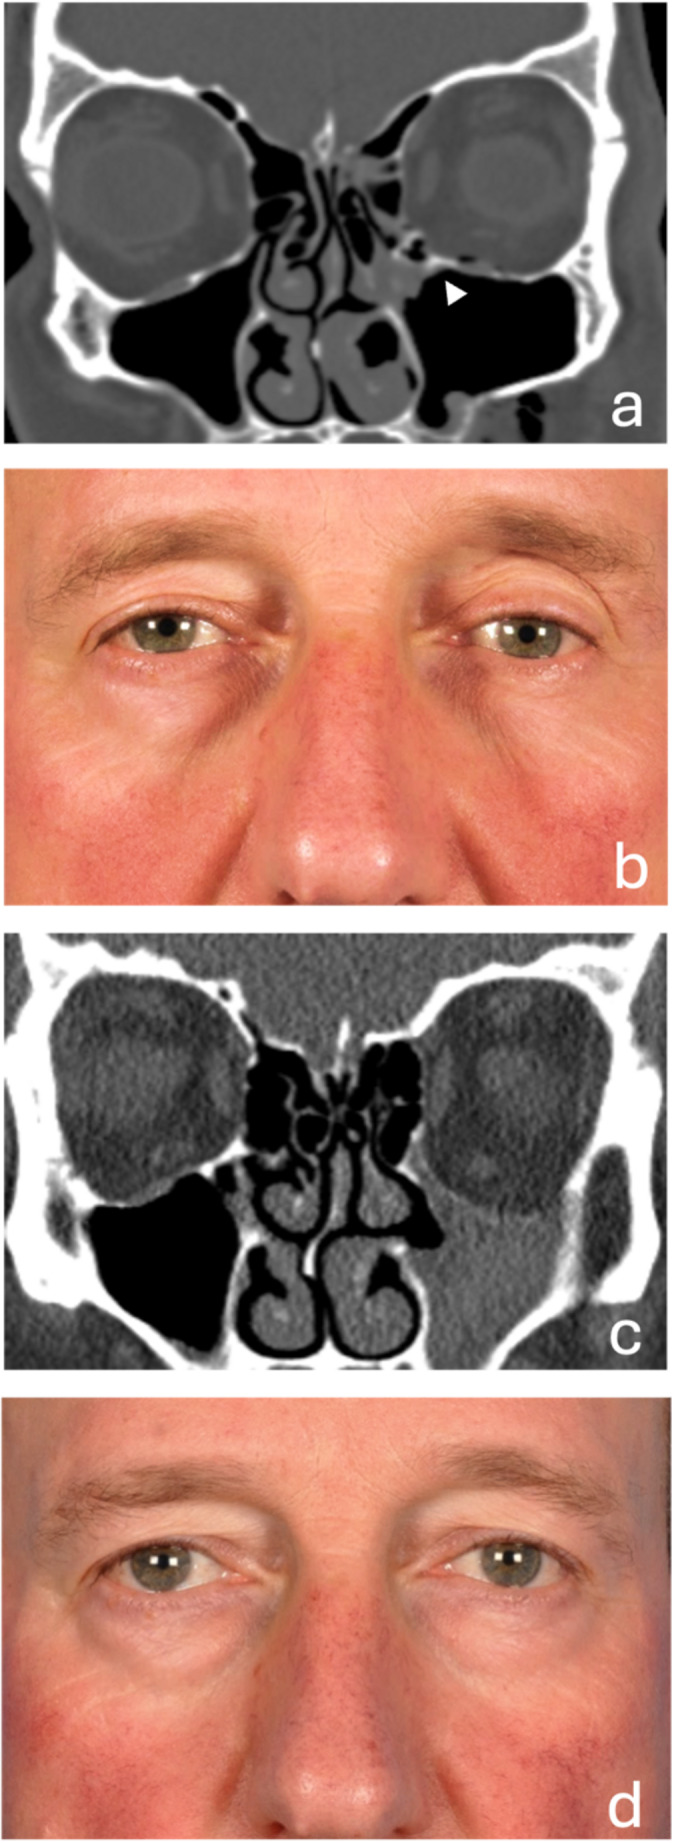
Posttraumatic secondary silent sinus syndrome (SSS). Note: A 58-year-old male patient following facial trauma. (A) Coronal CT at T1 (time of trauma). The scan shows a left zygomatic fracture involving the orbital floor and maxillary ostium region (arrow). Opacification of the infundibulum is noted, but no other signs of sinus pathology are evident at this stage. (B) Clinical photograph, twenty-one months post-trauma. There is a visible asymmetry in the upper eyelid sulci and light reflection on both corneas, consistent with enophthalmos and hypoglobus in the left eye. The patient reports diplopia on upgaze, which emerged six months after the trauma. (C) Coronal CT scan at T2. Signs of secondary SSS are evident, including complete opacification of the left maxillary sinus, lateralization of the left uncinate process, and depression of the orbital floor. (D) Clinical photograph, eleven months post-surgery. Following endoscopic retrograde uncinectomy and orbital reconstruction with a patient-specific PEEK implant. Diplopia is no longer present, and the left eye has been restored to a normal globe position, confirmed by Hertel measurements (12-118-12)
